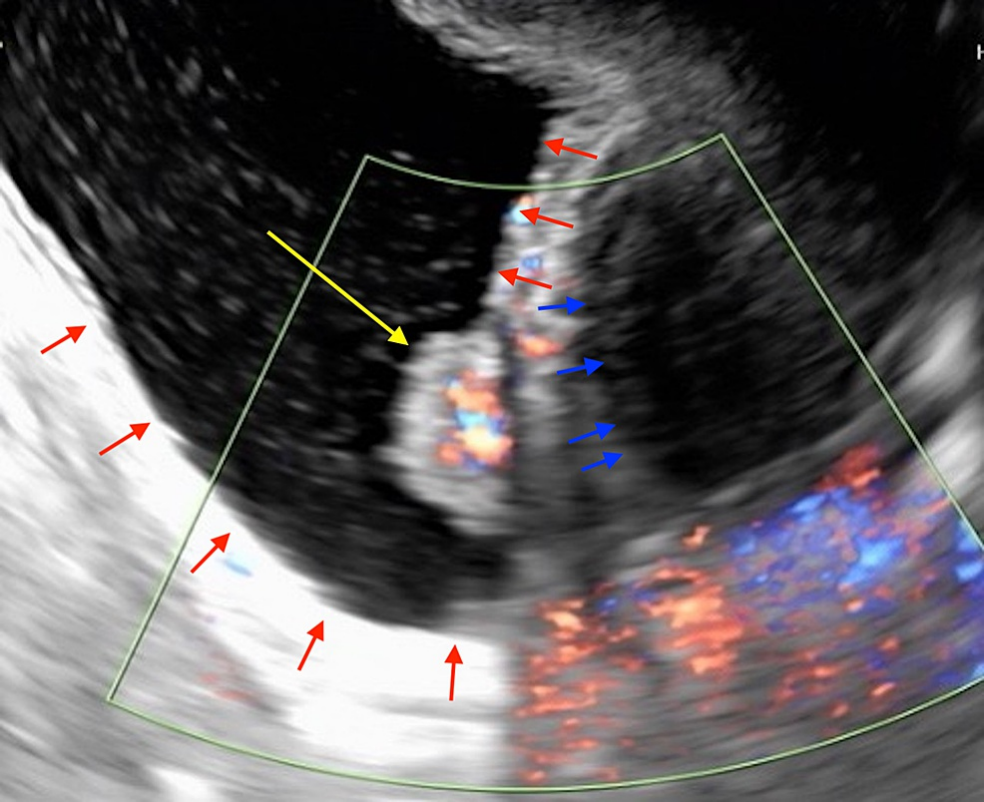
Transvaginal ultrasound image Yellow arrow: bladder tumor; red arrow: bladder; blue arrow: uterus A mass in the bladder mucosa by transvaginal ultrasound was observed.
